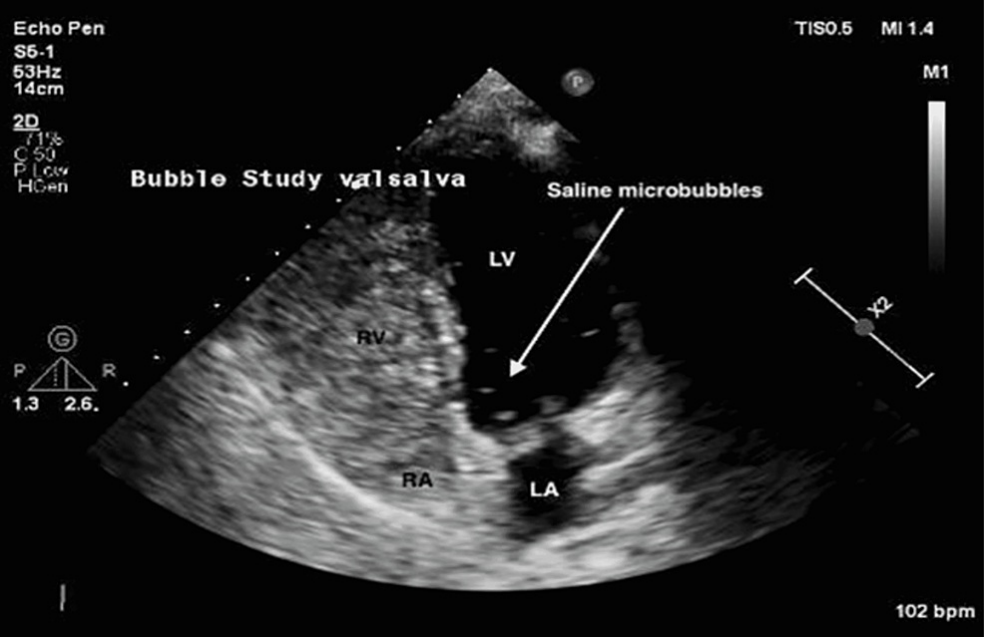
Transthoracic echocardiogram with bubble study pre-procedure. Transthoracic echocardiogram with bubble study during the relaxation phase of the Valsalva maneuver. The microbubbles seen in the left ventricle signify a right-to-left shunt and thus a positive test. Beats per minute (BPM), Left atrium (LA), Left ventricle (LV), Right atrium (RA), Right ventricle (RV).
